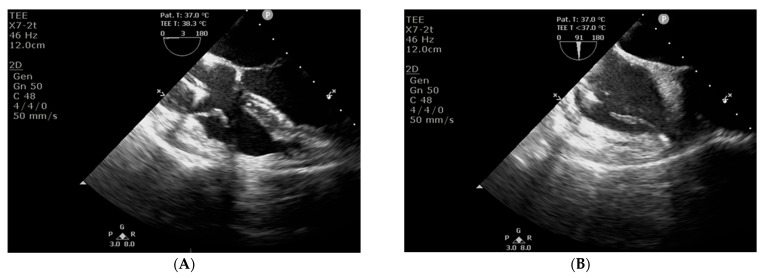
Transoesophageal echocardiography: structures consistent with vegetations on the leads (A, long-axis view) and in the right atrium (B, short-axis view).
